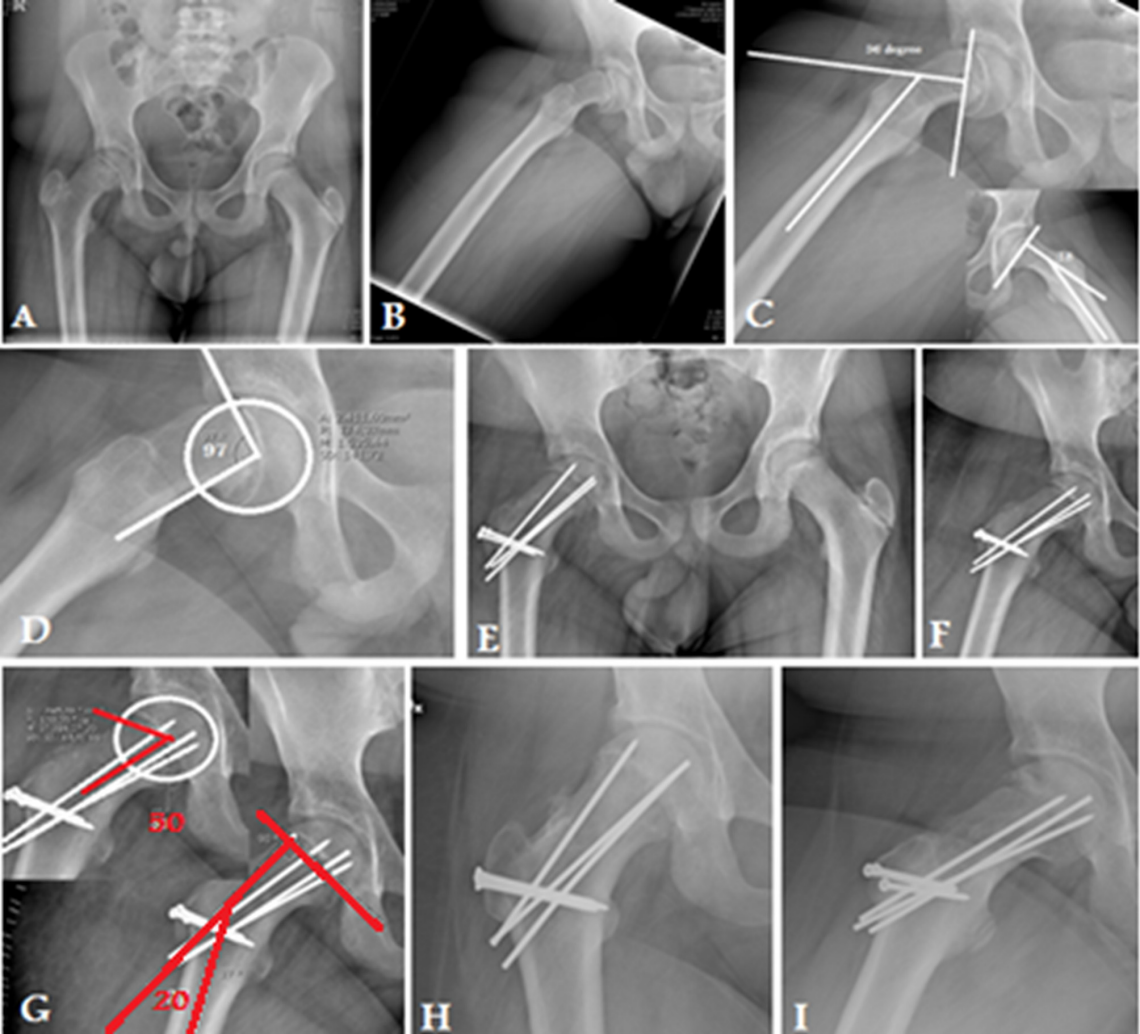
X-ray in a 15-year-old male with Rt chronic stable SCFE (case number 10): (A) preoperative AP pelvis, (B) preoperative frog lateral view of the Rt hip, (C) preoperative measurement of Southwick slip angle, (D) preoperative measurement of alpha angle, (E) and (F) postoperative anteroposterior (AP) and frog view, (G) postoperative measurement of Southwick slip and alpha angles, (H) 16-month postoperative AP and frog lateral X-ray showing complete union of trochanteric osteotomy and physis with no evidence of AVN.
